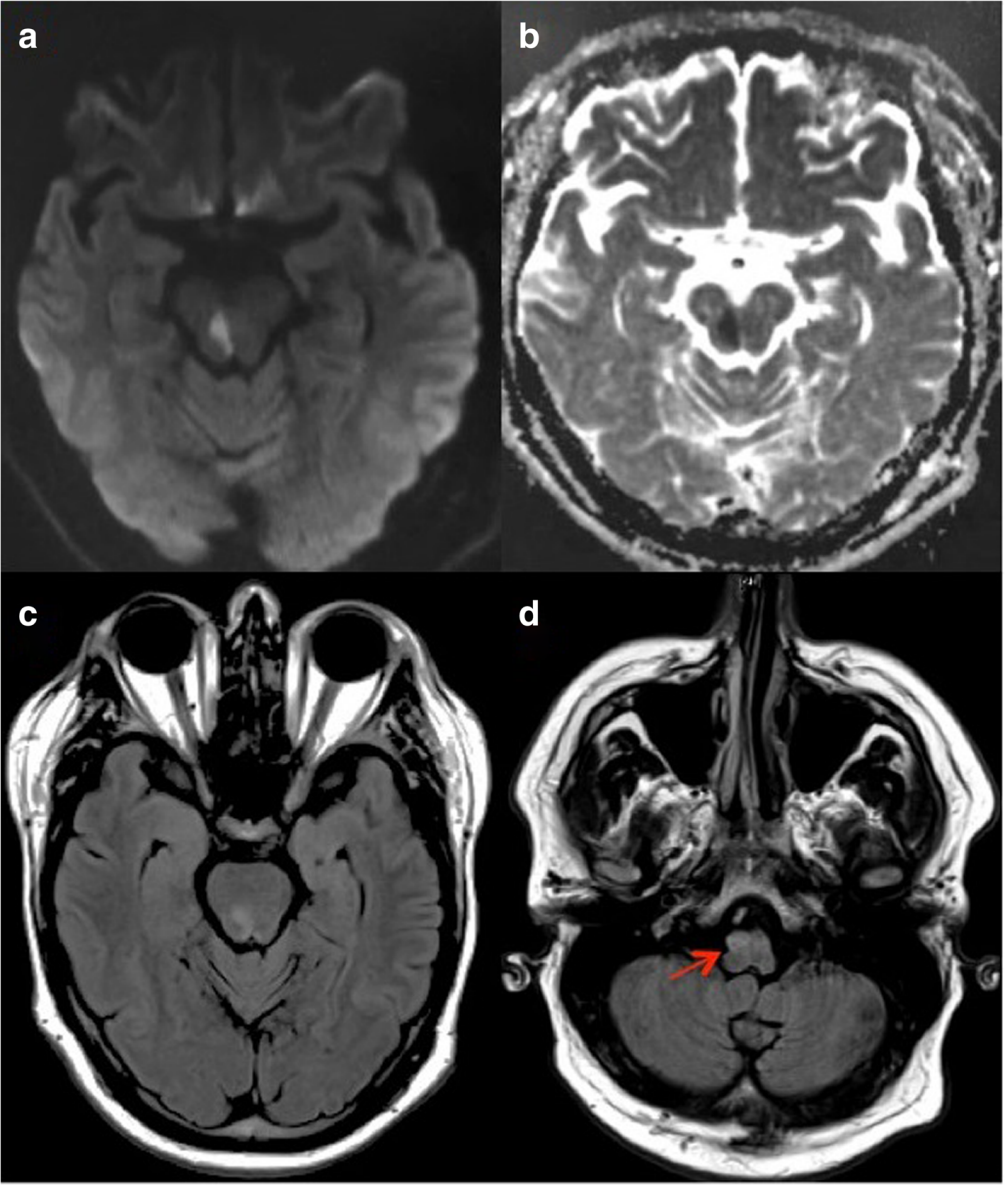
Brain magnetic resonance imaging axial sections. a and b Diffusion-weighted imaging and apparent diffusion coefficient sequences show diffusion restriction in the right caudal midbrain region. c and d T2-weighted fluid-attenuated inversion recovery sequences show hyperintensity in the right caudal midbrain with acute infarction and hypertrophic degeneration of the right olivary nucleus (*red arrow*)
